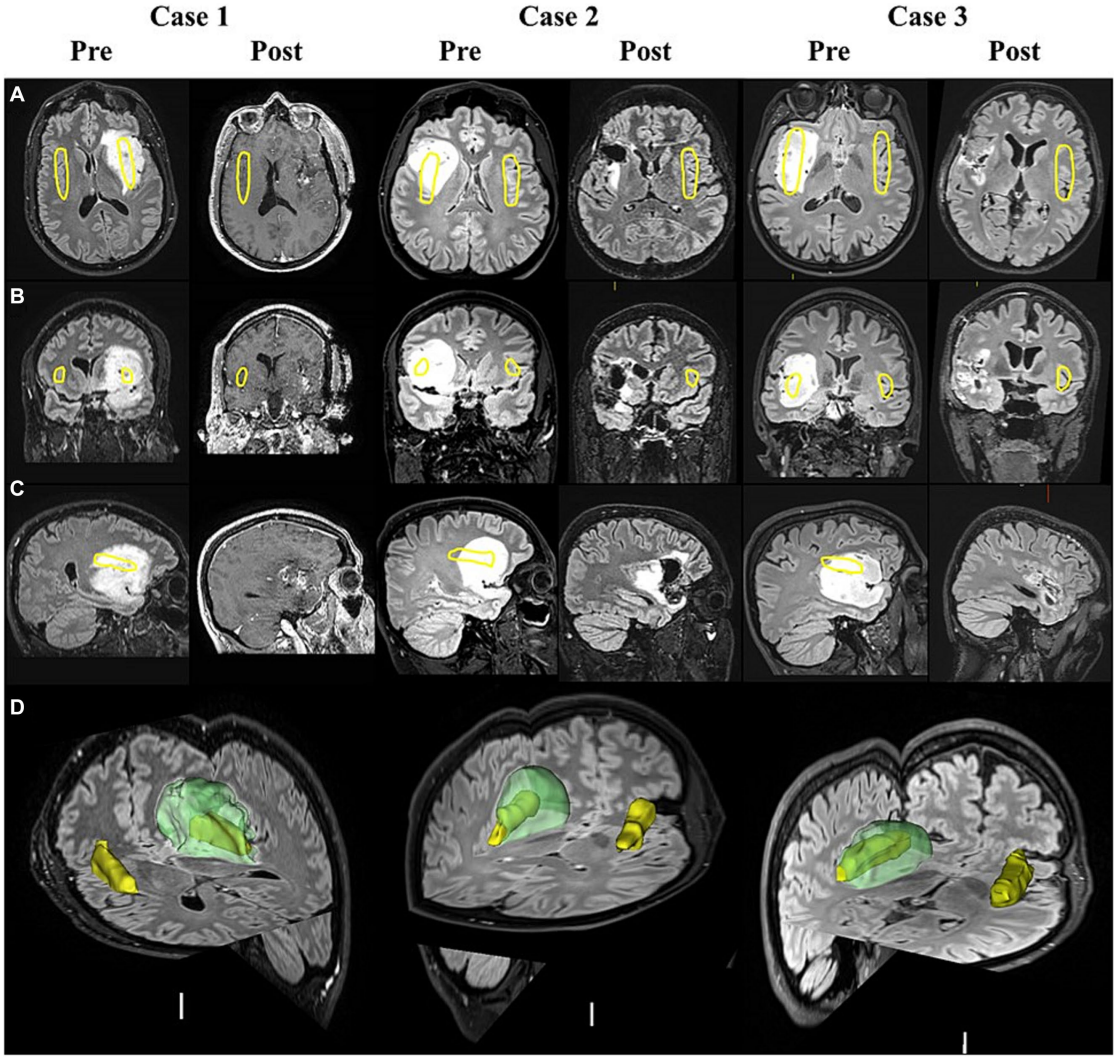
MRI scans of insula-glioma pre- and post-surgery. T2 weighted FLAIR MRI images displayed in the axial (A), coronal (B), and sagittal (C) planes before surgery (Pre) and after surgery (Post) for cases 1 to 3. Case-1 did not have a post-surgery FLAIR scan as they were isolating abroad during the COVID-19 pandemic and therefore available post-surgery T1 weighted MRI scans were used (48 months before post-surgery testing). Yellow outlines represent the positioning of the insular cortex. Panel (D) displays the segmented insula (yellow) and glioma (Green) generated using pre-surgery FLAIR scans in 3D slicer for each case.
